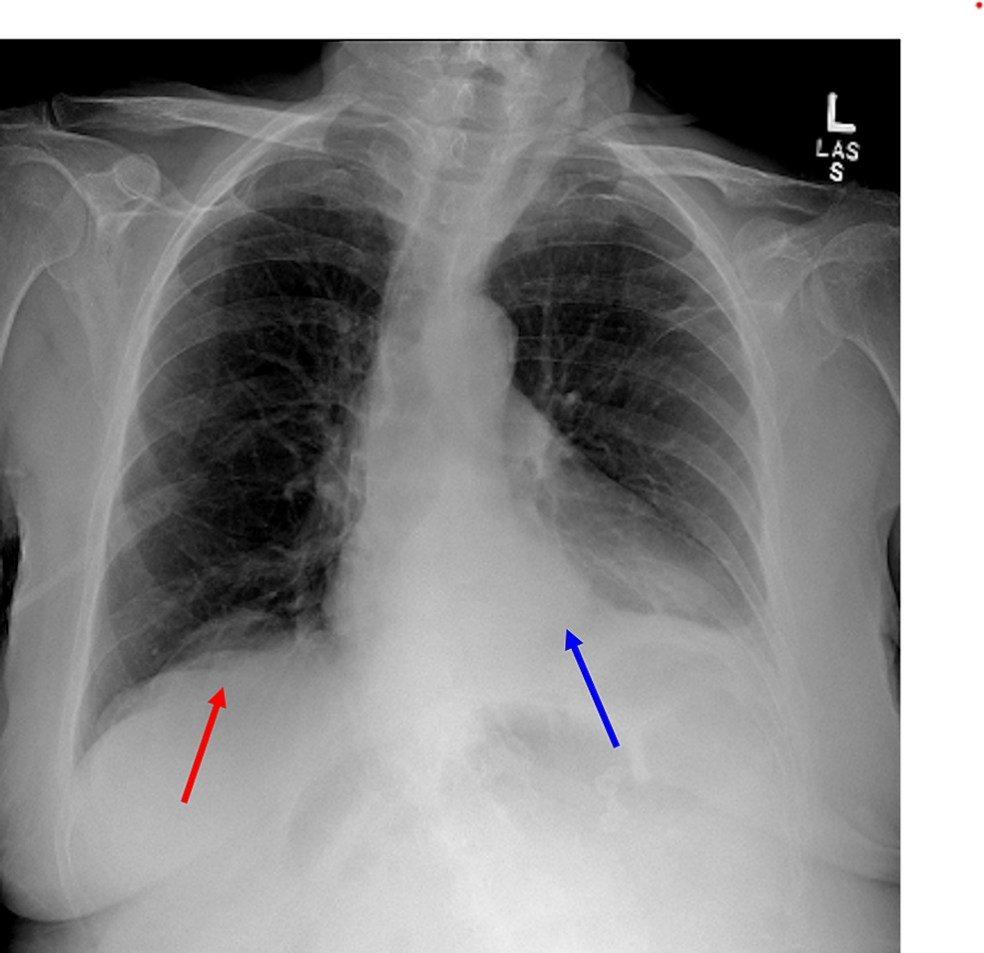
Chest radiography remarkable for a moderate size hiatal hernia (blue arrow), eventration of the right hemidiaphragm (red arrow), left basilar density with blunting of the left costophrenic angle, and scoliosis
